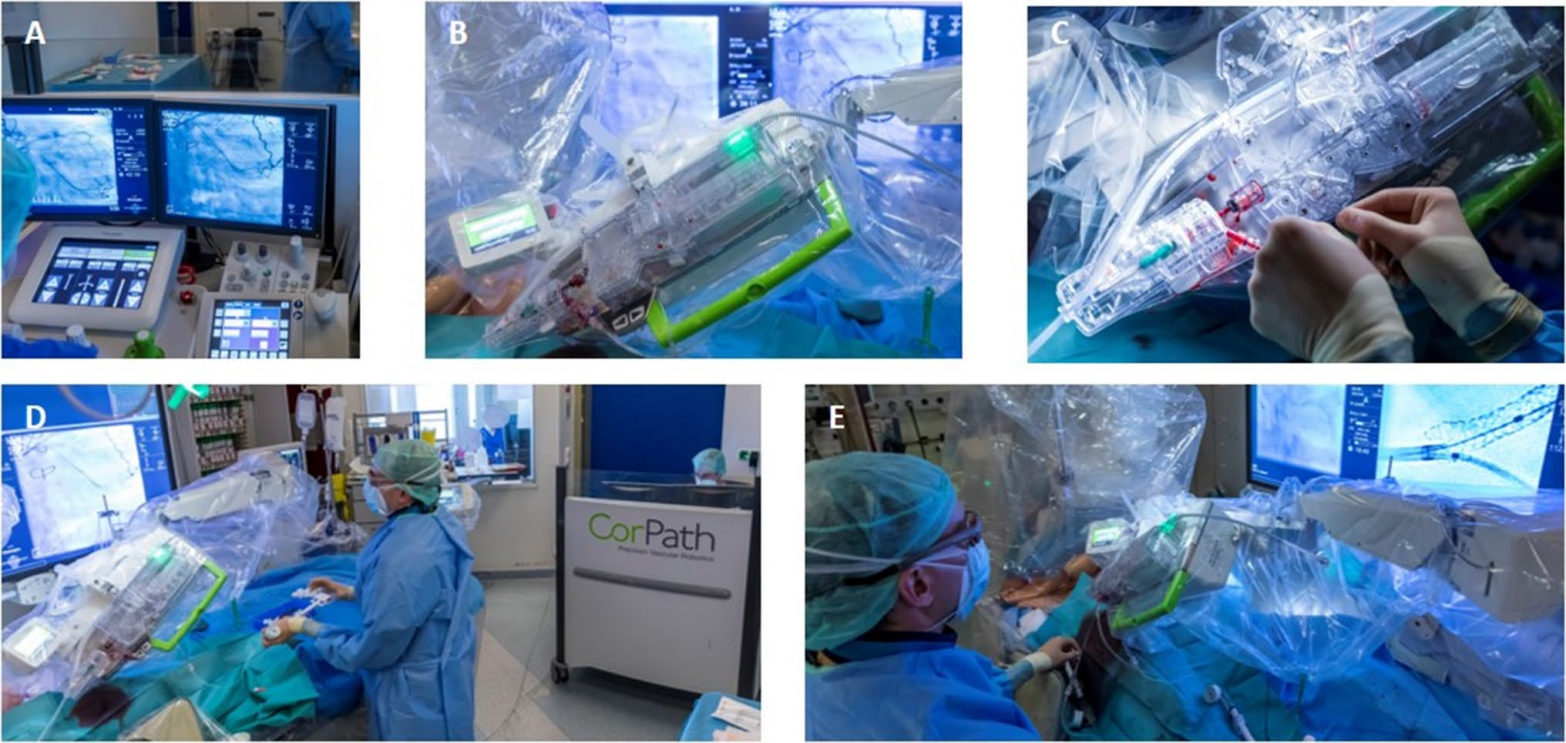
The rPCI platform and setup in the catheterization laboratory. The interventionalist’s cockpit features remote controls for the rPCI system, c -arm, X-ray tube, and radiation protection shields (A). The robotic arm, housing the disposable cassette, after setup and connection to the sheath and guiding catheter enabled for robotic-assisted navigation (B). Exchange of wires and devices by the assistant is performed at the patient’s bedside (C). The setup allowed for a distance between the assistant and the c-arm to reduce X-ray exposure (D). The “hybrid approach” was utilized for bifurcation stenting to accelerate the procedure: the second balloon or stent was guided manually by the second operator while the first device was navigated robotic-assisted (E)
